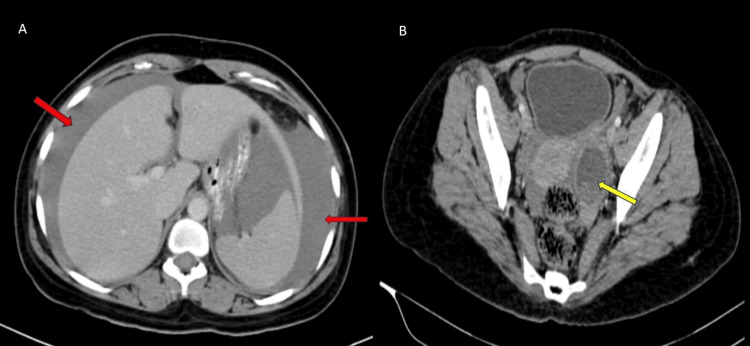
CECT of the abdomen and pelvis showing (A) hypodense fluid in the abdomen and pelvis suggestive of hemoperitoneum (red arrow) and (B) well-defined peripherally enhancing cystic lesion in the left ovary with the hyperdense non-enhancing component within the cyst suggestive of the hemorrhagic cyst (yellow arrow). CECT, contrast-enhanced computed tomography
